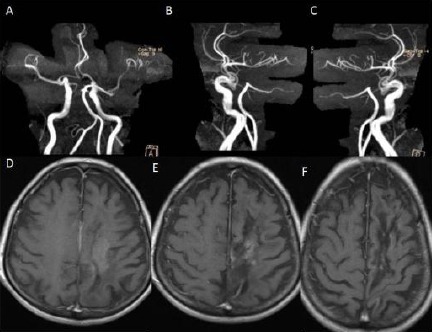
A, B, C - Cranial MR angiography showing no damage any arterial structures due to the biopsy procedure. D, E, F - Cranial MRI showing complete resorption of the hematoma 7 months later of the operation*.
